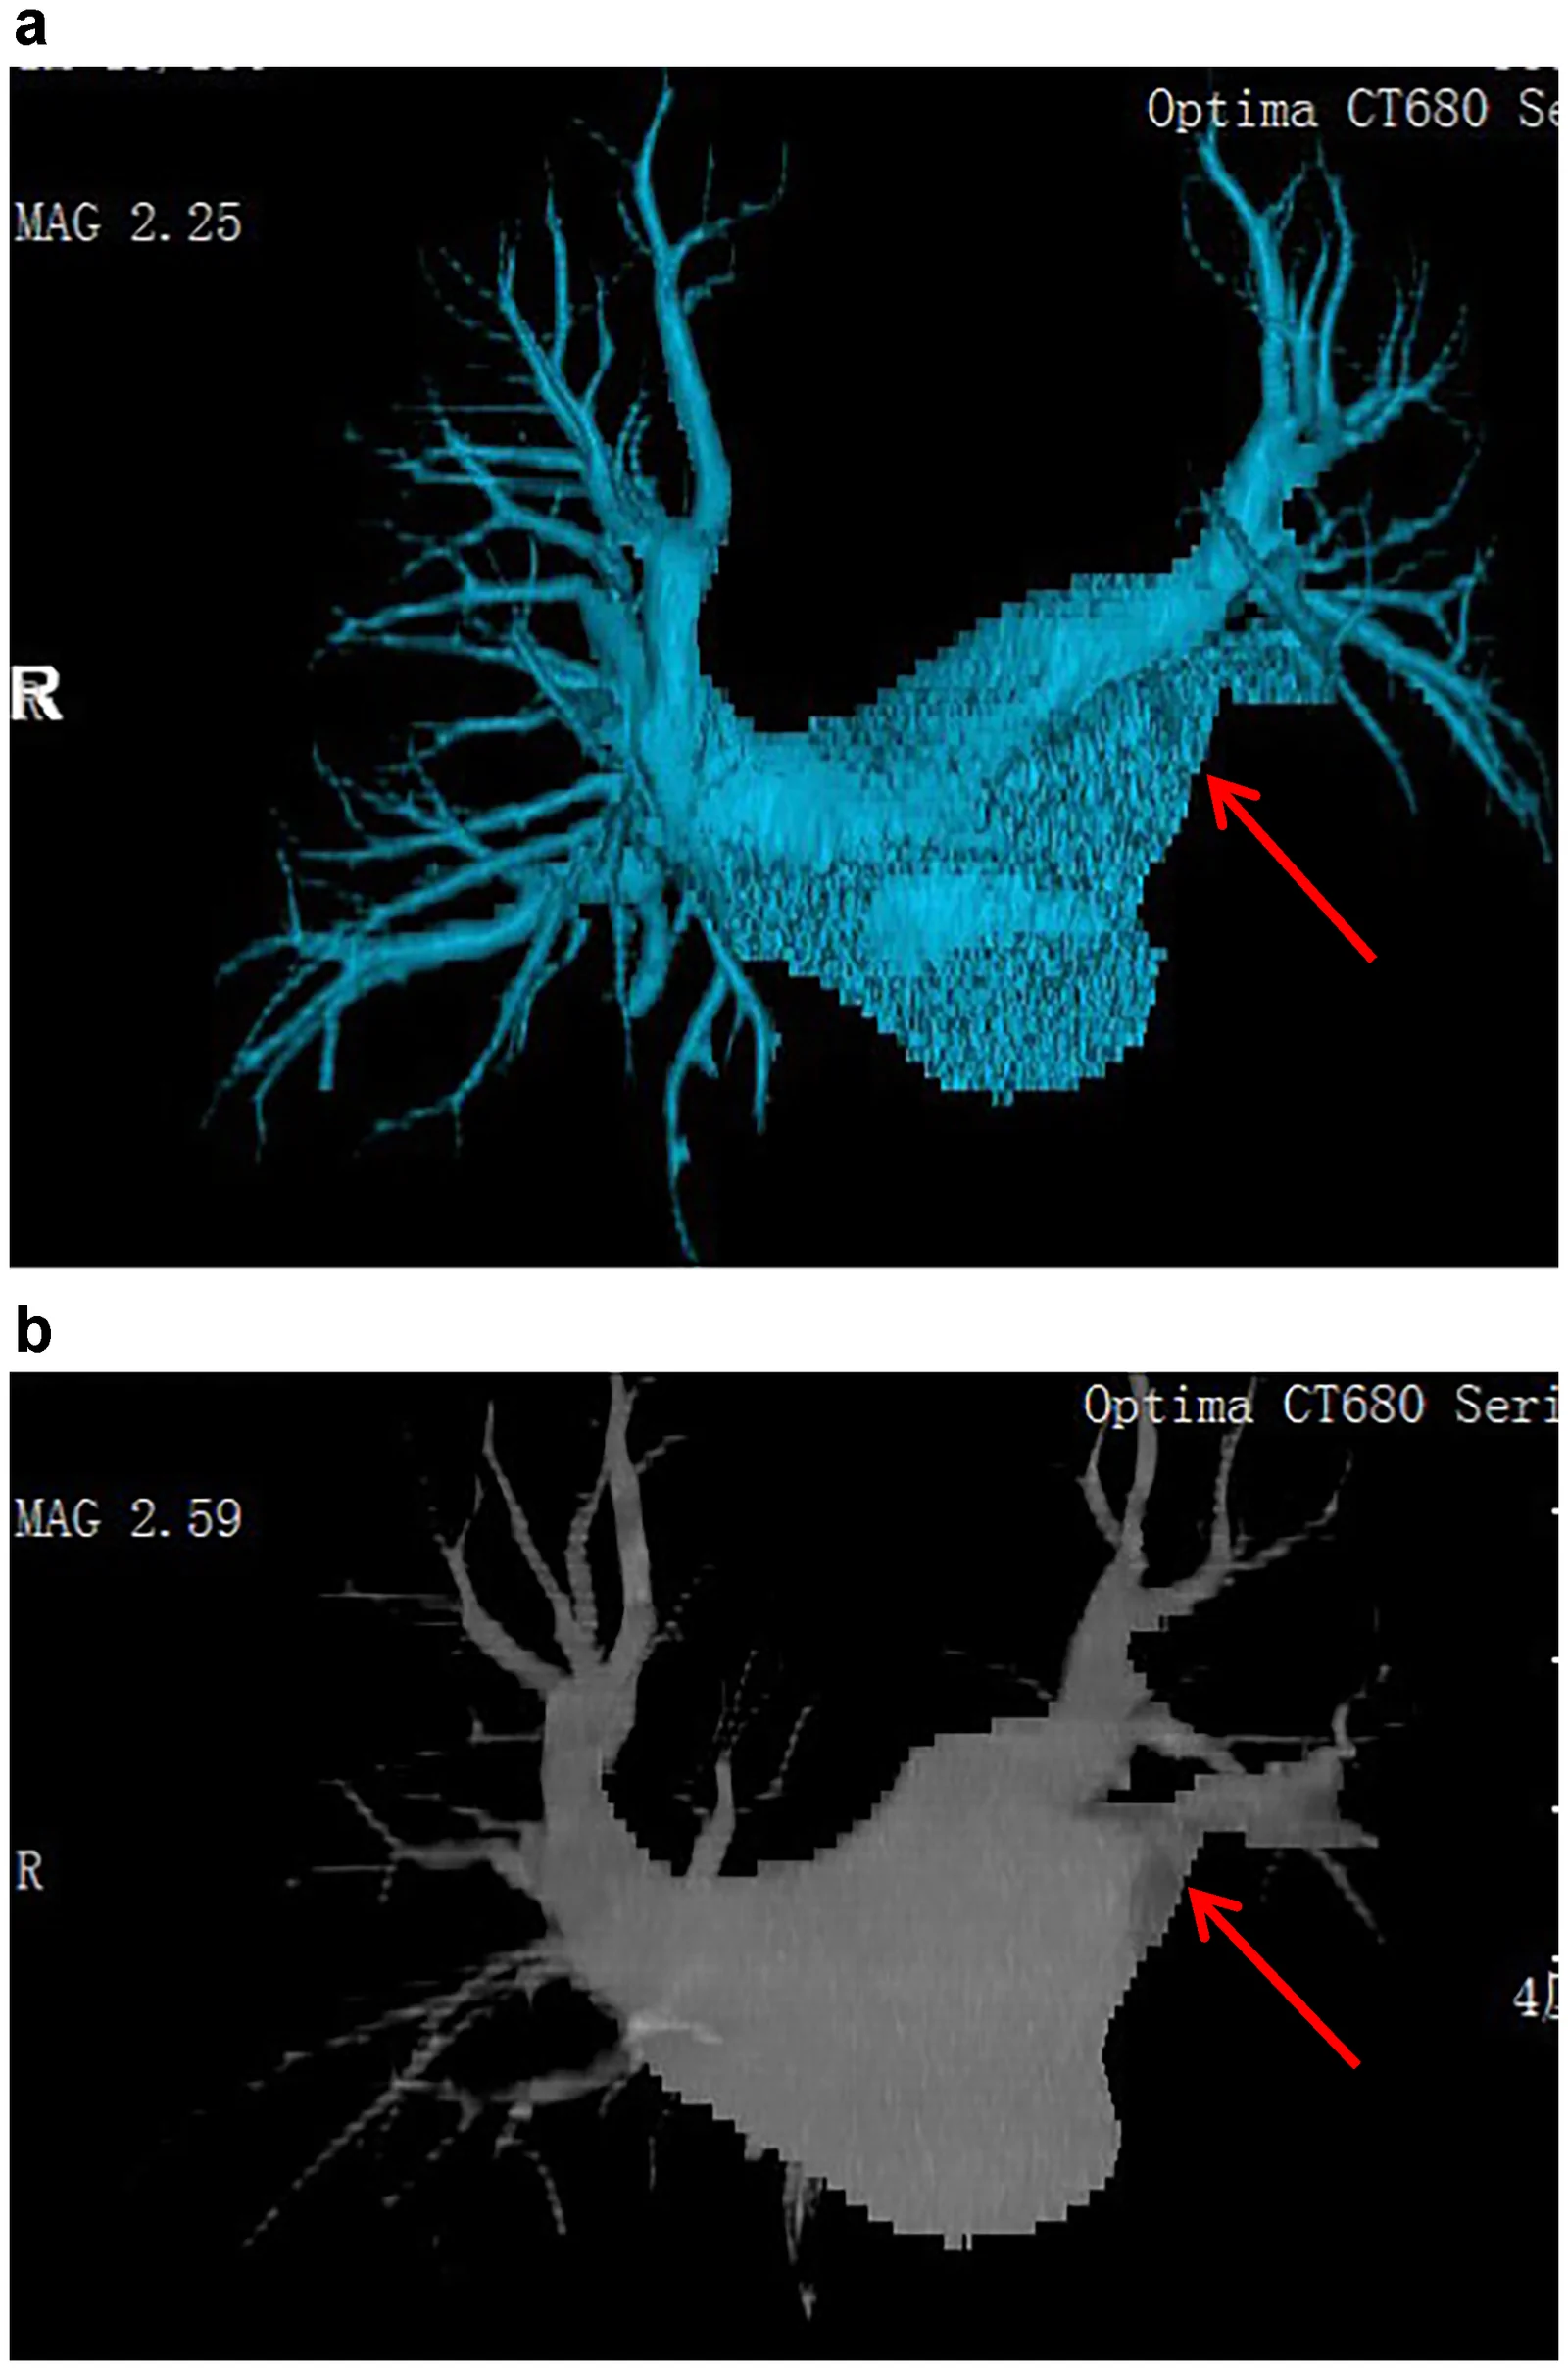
Computed tomography angiography. (a) Volume rendering image of the pulmonary veins. (b) Maximum intensity projection image of the pulmonary veins. A filling defect shadow was visible in the left inferior pulmonary vein (red arrow).
